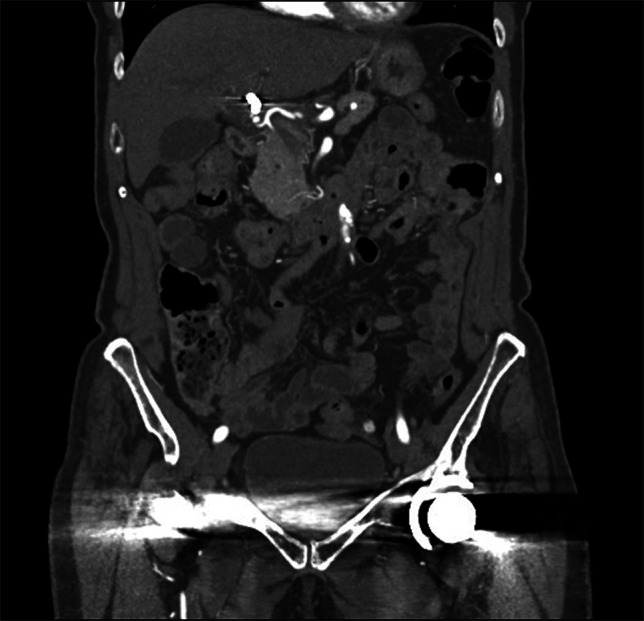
Coronal view of postembolization abdominal computed tomography with left hepatic artery pseudoaneurysm, with coils in place.
